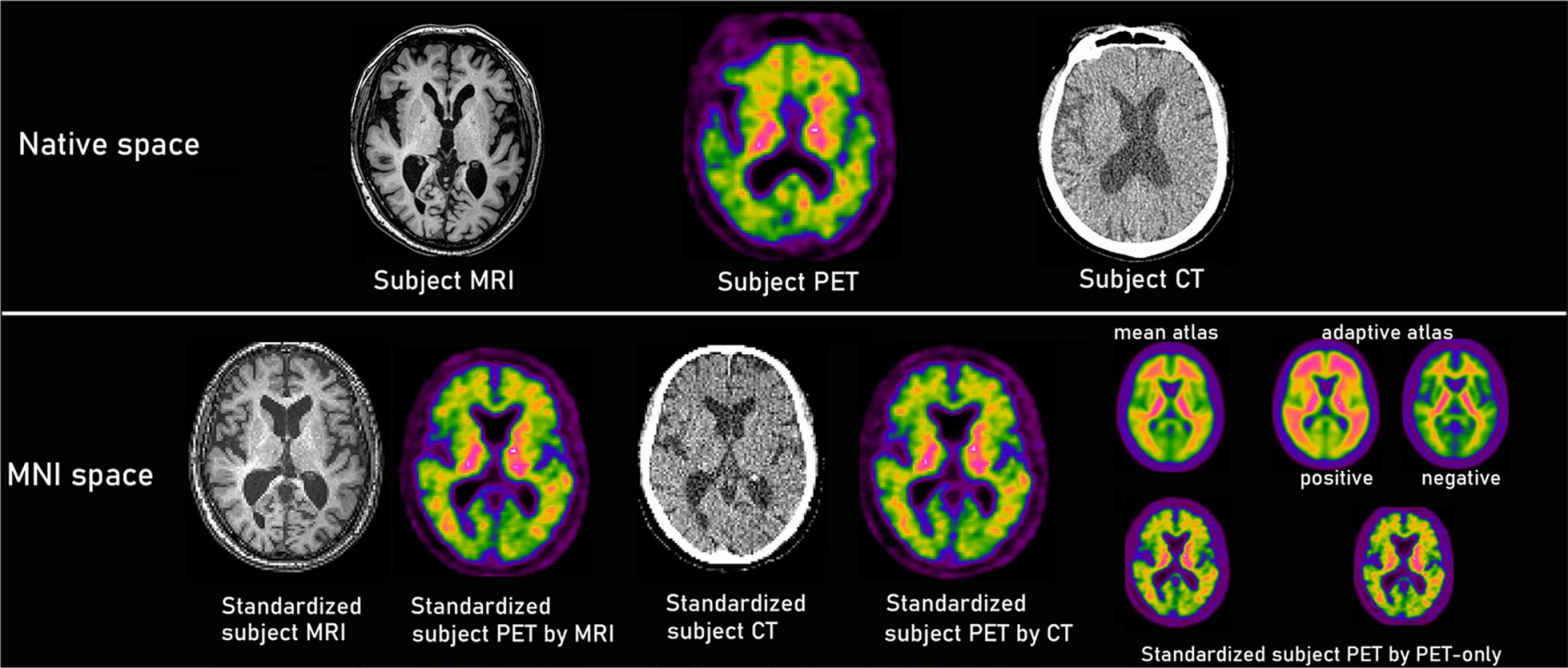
Coregistered (upper row, native space) and anatomically standardized (lower row, MNI space) MRI, PET, and CT images. Almost identical PET images were obtained after anatomic standardization between the MRI-based and CT-based approaches. The PET-only approach involved anatomic standardization using a mean atlas for a single template or an adaptive atlas for a positive or negative template
